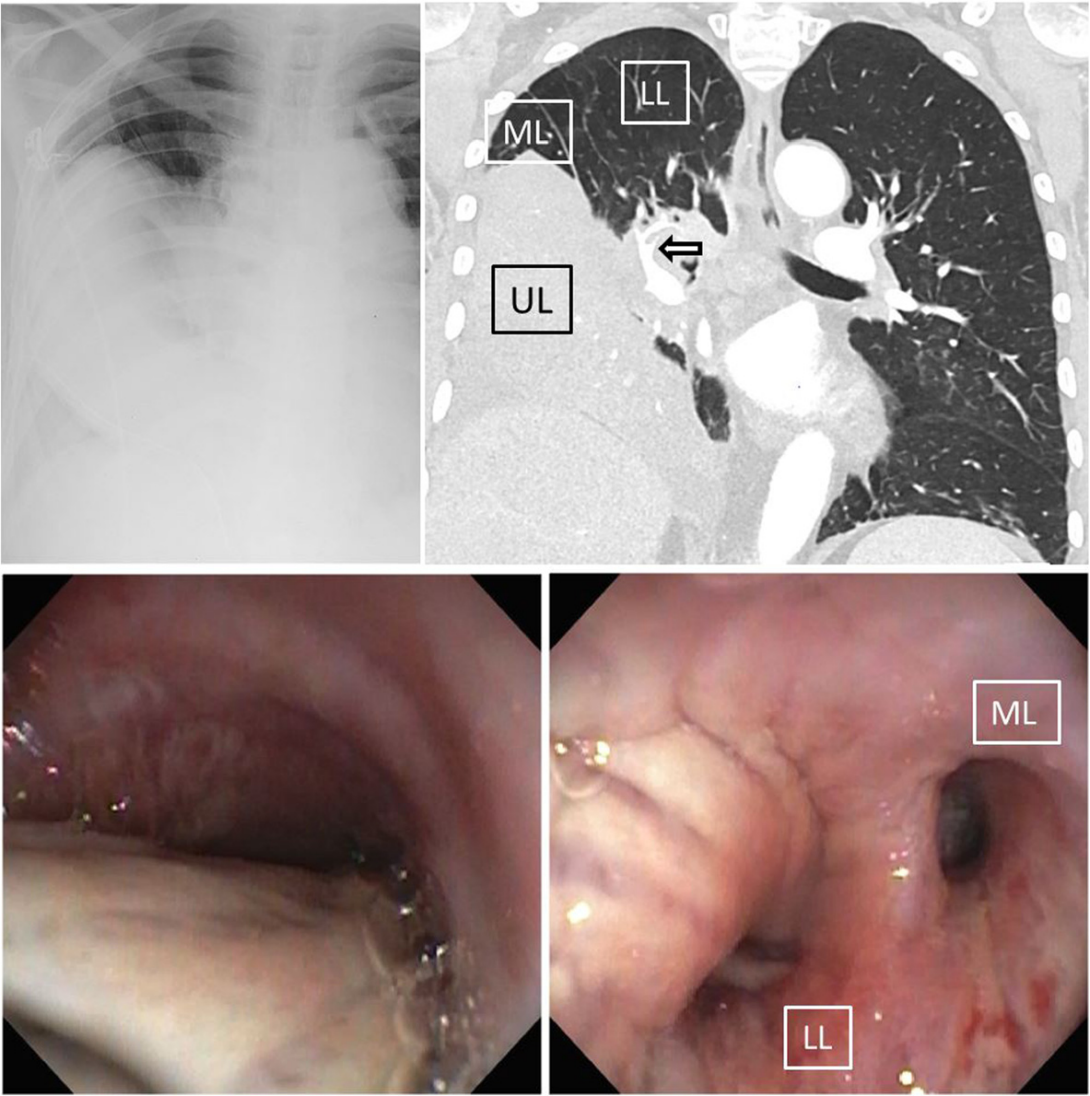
Upper images: Chest X-ray showing right sided lung atelectasis and pleural effusion. On the right: Chest-CT showing downward rotation of the congested and completely atelectatic upper lobe (UL) to the base of the chest cavity with concomitant upward shift of the middle (ML) and lower lobe (LL). Also note the upward torsion of the pulmonary artery to the right lower lobe resulting in a typical ‘whirl sign’ (*arrow*). Lower images: Brochoscopy showing compression/torsion of the right mainstem bronchus (*left image*). Whereas the upper lobe bronchus was completely obstructed, the middle (ML) and lower lobe bronchus (LL) showed rightward torsion resulting in partial obstruction of the corresponding lobes (*right image*)
